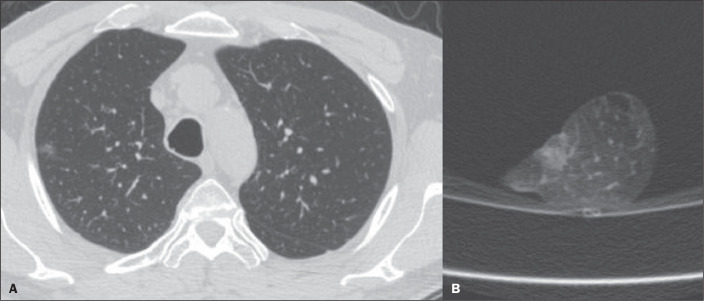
A: Axial CT scan of a 41-year-old male patient revealed a subsolid
